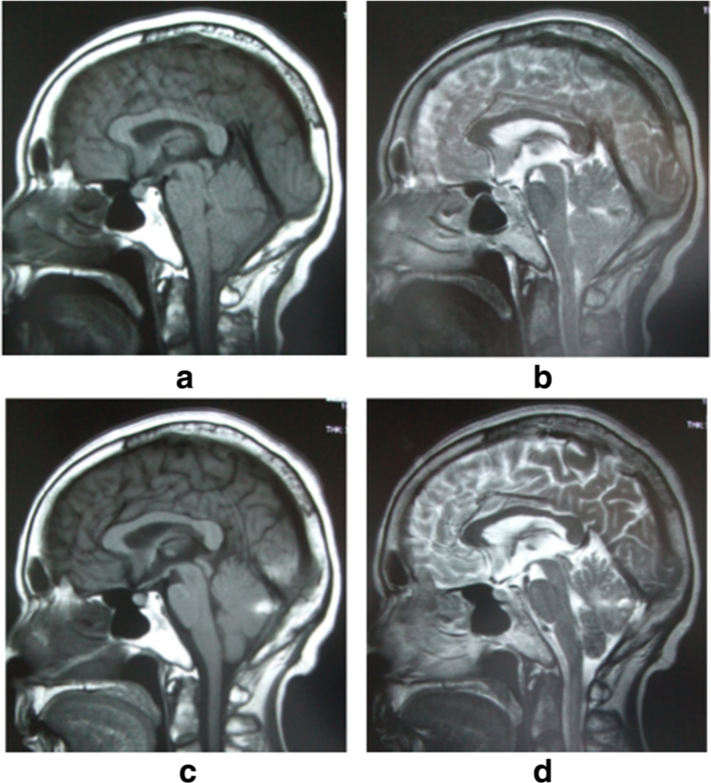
Sagittal magnetic resonance imaging taken 13 hours and 12 days after spinal surgery. Sagittal T1-weighted (a) and T2-weighted (b) magnetic resonance images taken 13 hours after spinal surgery, demonstrating herniation of the cerebellar tonsils. Sagittal T1-weighted (c) and T2-weighted (d) magnetic resonance images taken 12 days after spinal surgery demonstrating ascent of the cerebellum to the normal position.
